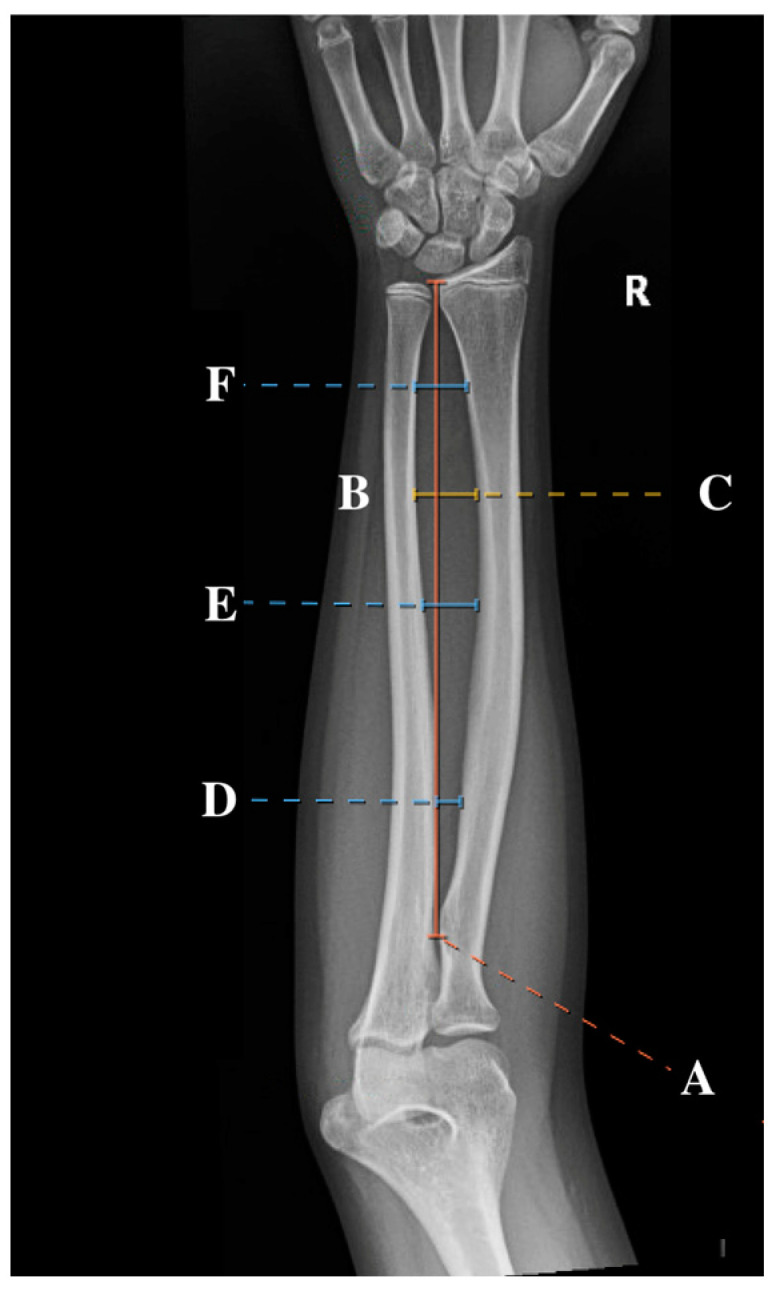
An AP radiographic view of the forearm of a 12-year-old female with the six measurements included in this study: total radial length (A), location of the radial bow (B), maximum radial bow (C), and proximal (D), middle (E), and distal (F) radial bow.
